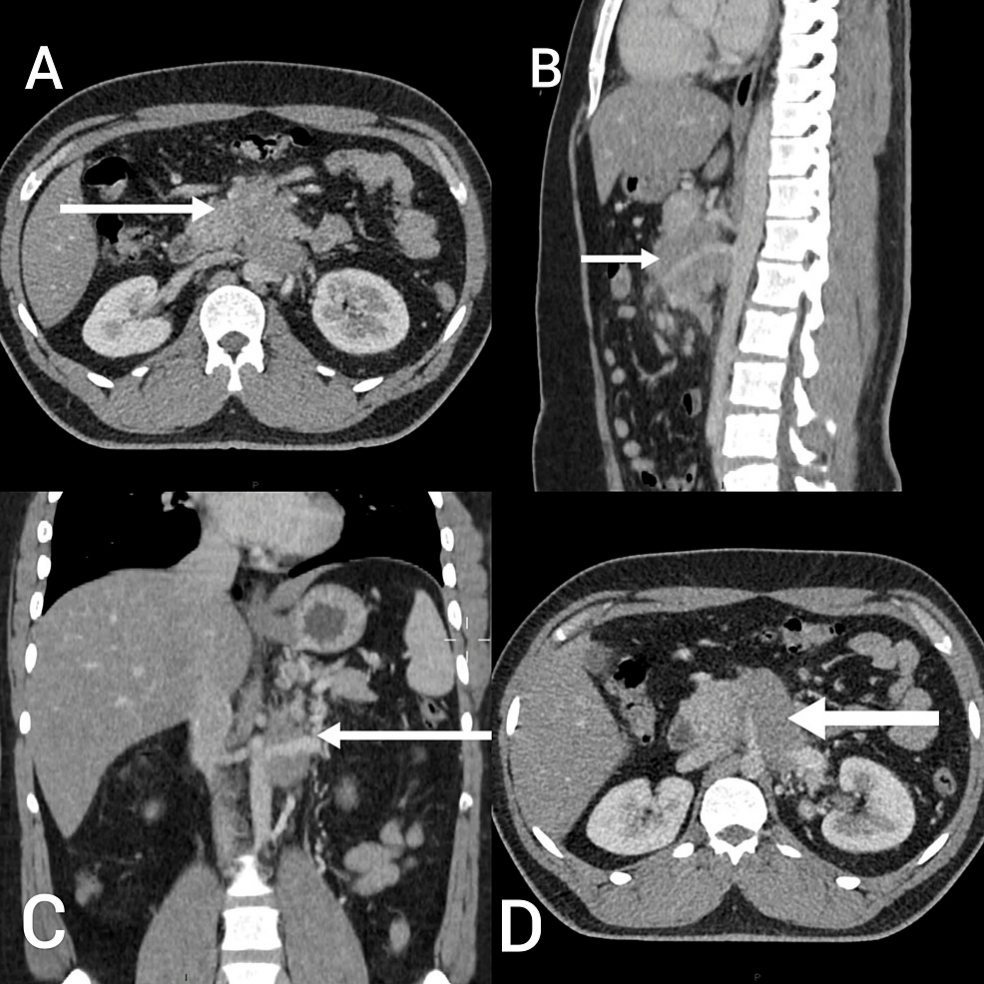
CT scan showing heterogeneously enhancing soft tissue density mass in retroperitoneum. Axial views showing the mass abutting the superior mesenteric vein anterolaterally (A) and inferior vena cava posteriorly (D). Lateral view showing the mass encasing the superior mesenteric artery, loss of fat planes, and inferior vena cava posteriorly (B). Frontal view showing the mass encasing the left renal vein (C).
